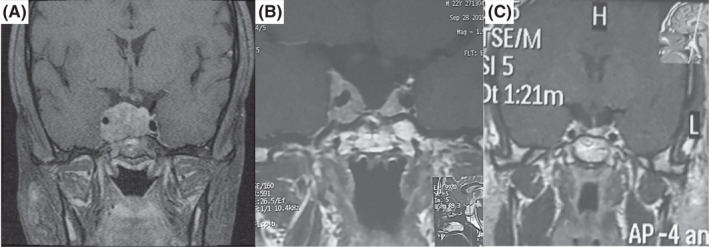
(A) Coronal images of pituitary gland MRI, T1‐weighted post‐contrast demonstrate sellar mass with suprasellar and right cavernous sinus extension. (B) Post‐second surgery and radiation study shows a marked decrease in mass size with residual tumor along the cavernous sinus. (C) After TMZ treatment , there is a near‐complete response
